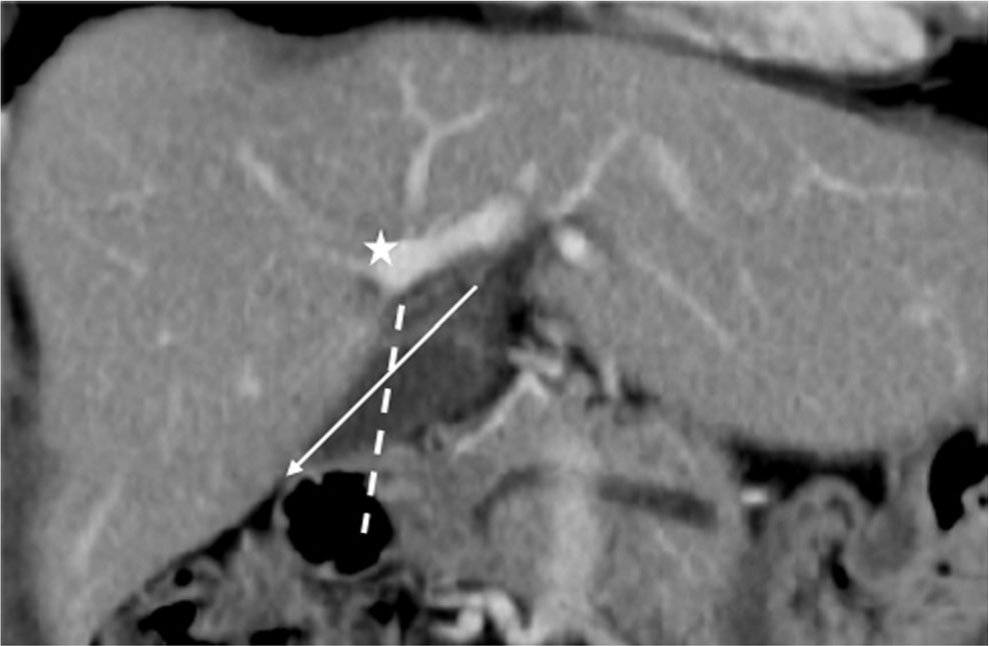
Contrast-enhanced CT revealing the gallbladder with a normal cholecystic axis (*arrow*) to the right of the umbilical fissure (*star*) and RSLT (*dotted line*)
